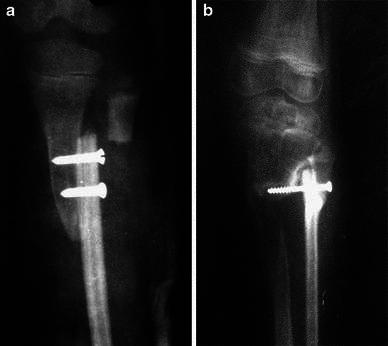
a Radiograph of an 8-year-old child showing proximal fixation of the fibular graft using two screws. b Radiograph of a 9-year-old child showing proximal fixation of the fibular graft using one screw
